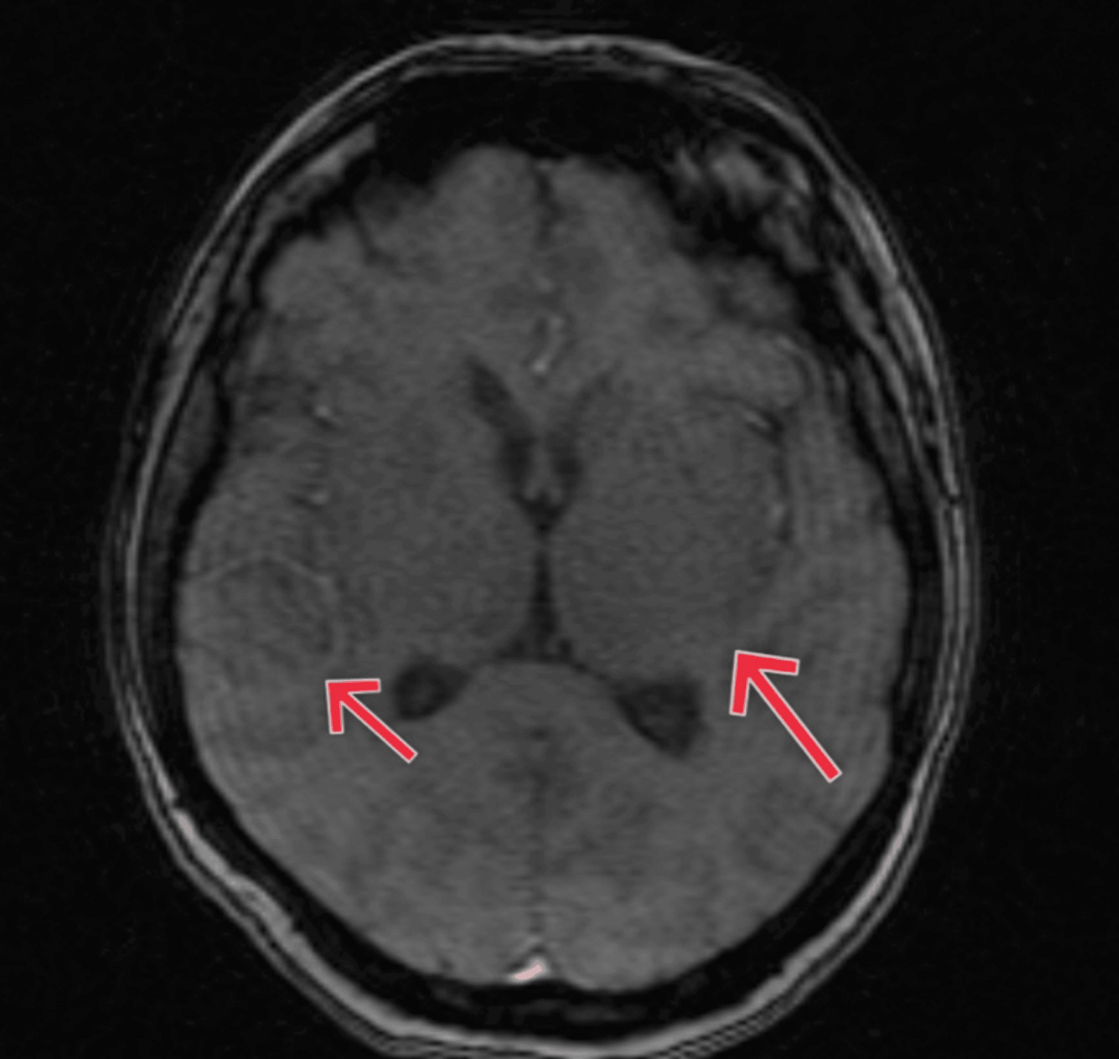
Axial fluid-attenuated inversion recovery (FLAIR) MRI showing bilateral hyperintense lesions in the basal ganglia (lentiform nuclei), consistent with edema.
